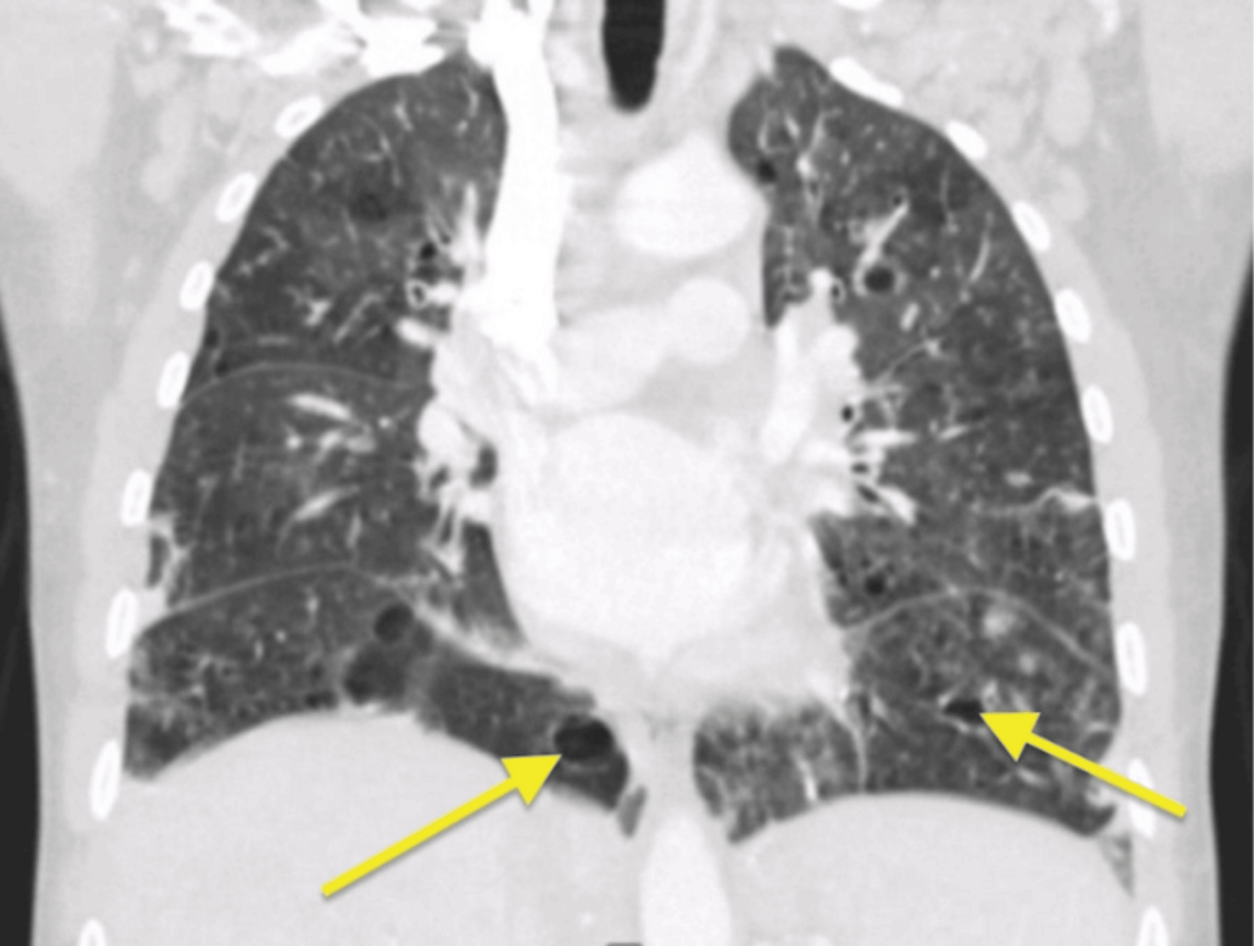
CT scan of the chest showing diffuse cystic lesions (yellow arrows).
